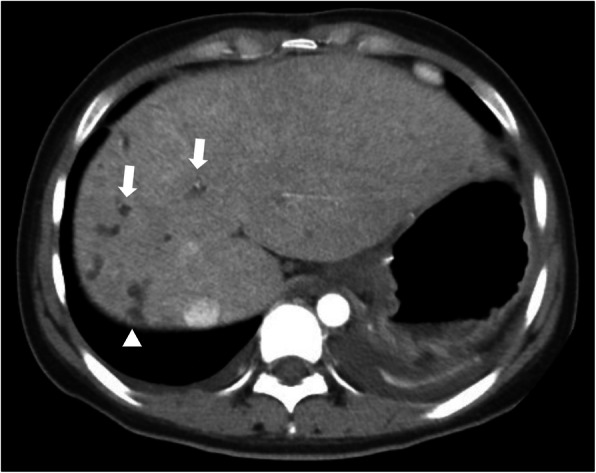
CT scan of a CS patient revealing multiple dilatations of intrahepatic bile ducts (arrow) and tiny dots of strong contrast within, which is the central dot sign (arrowhead)
